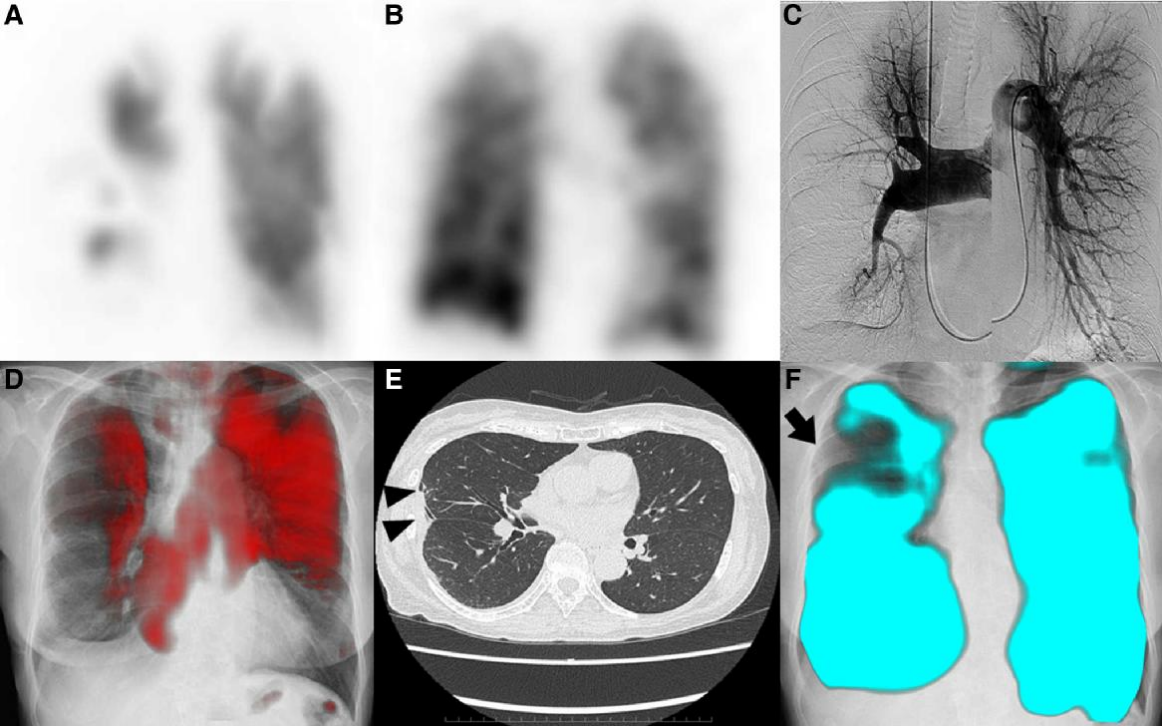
(*A*, *B*) Pulmonary perfusion/ventilation scintigraphy. (*C*) Pulmonary angiography. (*D*) Dynamic chest radiology to evaluate pulmonary perfusion. (*E*) High-resolution computed tomography. (*F*) Dynamic chest radiology image visualizing pulmonary expansion.
